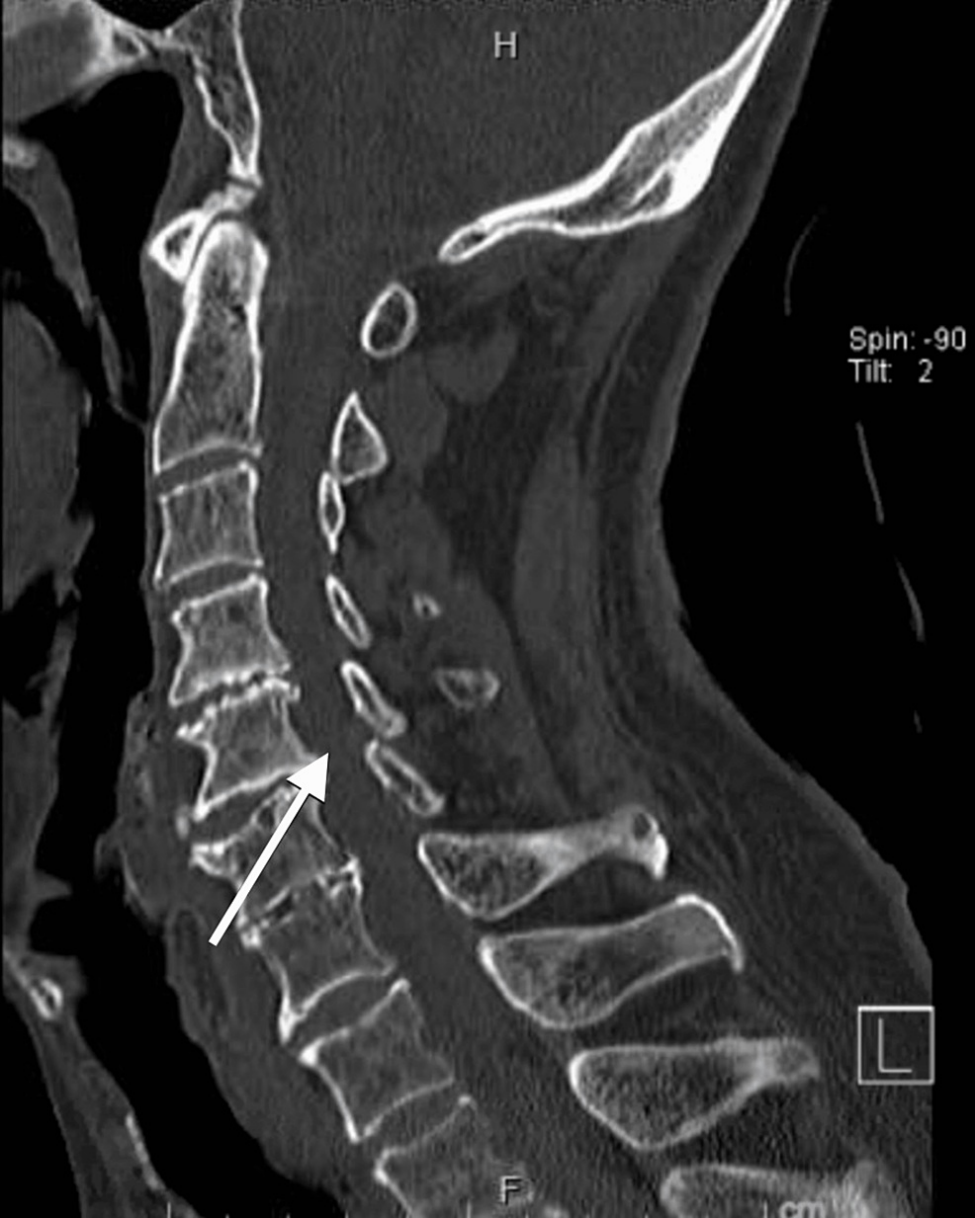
Computed Tomography of Cervical Spine Computed tomography (CT) scan of the cervical spine showing evidence of significant stenosis at C5-C6 level (arrow).
